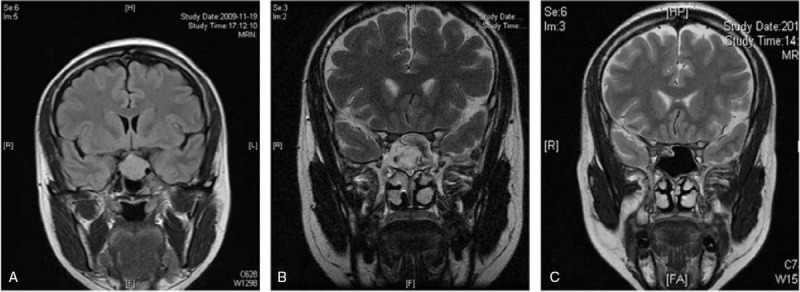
Coronal magnetic resonance imaging of the head of a female patient, who underwent resection of the transsphenoidal pituitary adenomas (A) before surgery; (B) 3 months after surgery and before treatment; (C) 47 months after surgery and 44 months after treatment.
